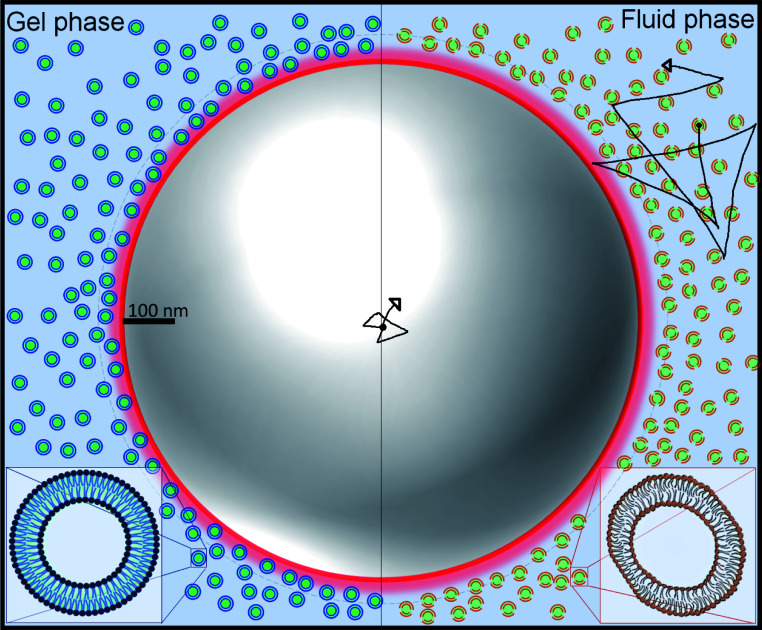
Graphical representation of SUVs and a tracer. The left (right) part of the image represents the gel (fluid) phase of the vesicles, respectively. In the gel (fluid) phase, the vesicles are rigid (soft and deformable). Note the much larger size of the tracer particle compared to the small size of vesicles.
